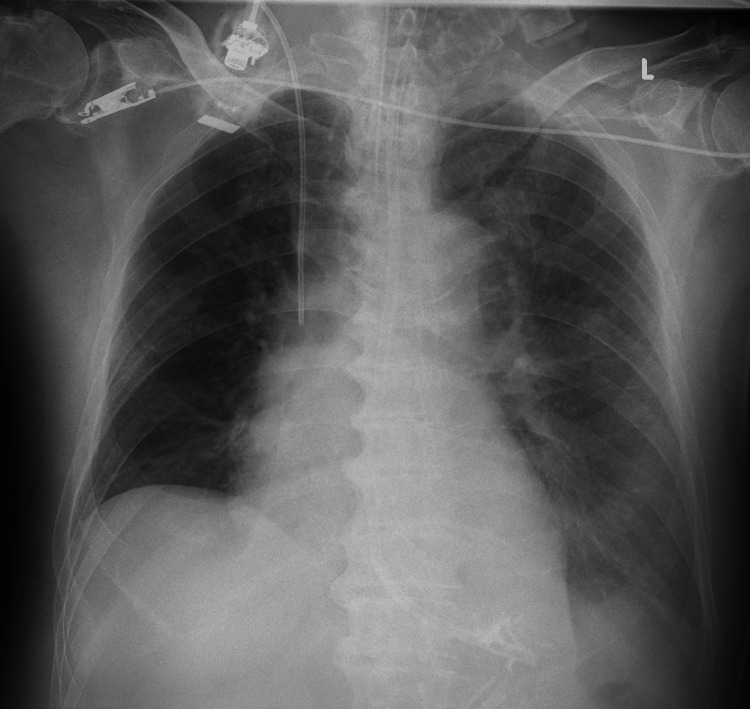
Chest X-ray showed pulmonary edema and pleural effusion on the fifth day of admission
